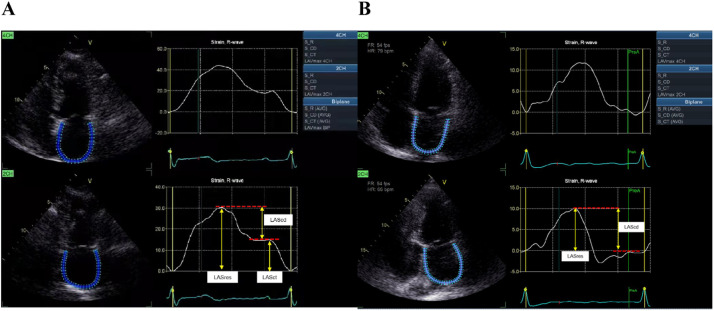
LA strain measurement acquired in the apical 4- and 2-chamber views by 2D-STE in a patient with sinus rhythm (A) and AF (B). LASres, Left Atrial reservoir Strain; LAScd, Left Atrial conduit Strain; LASct, Left Atrial contractile Strain.
